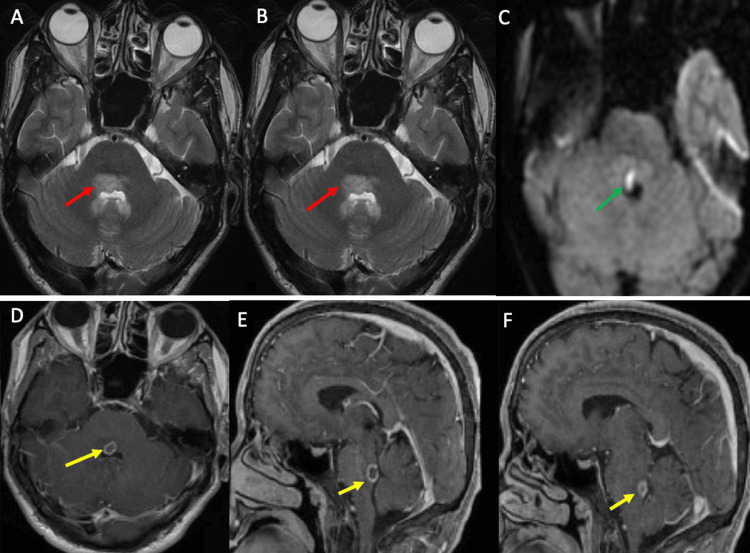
Brain MRI findings (A, B) Axial and sagittal T2WI show a 9 × 8 mm hyperintense pontine lesion located anterior to the fourth ventricle (red arrows). (C) DWI shows marked diffusion restriction within the lesion (green arrow). (D-F) Post-contrast T1WI demonstrate peripheral ring enhancement with posterior interruption at the ventricular interface (yellow arrows). MRI: magnetic resonance imaging; DWI: diffusion-weighted imaging; T1WI/T2WI: T1-/T2-weighted imaging.
